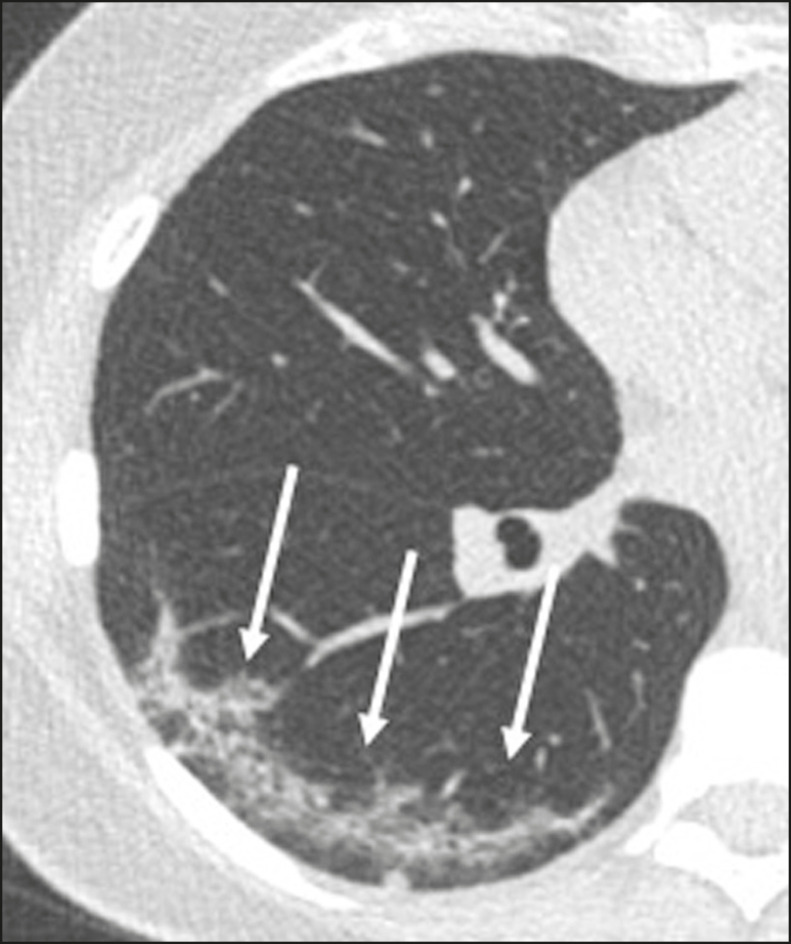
Chest CT scan showing a subpleural line (arrows) in a patient with a confirmed diagnosis of COVID-19 who presented with a 9-day history of dry cough, dyspnea, and anosmia.
